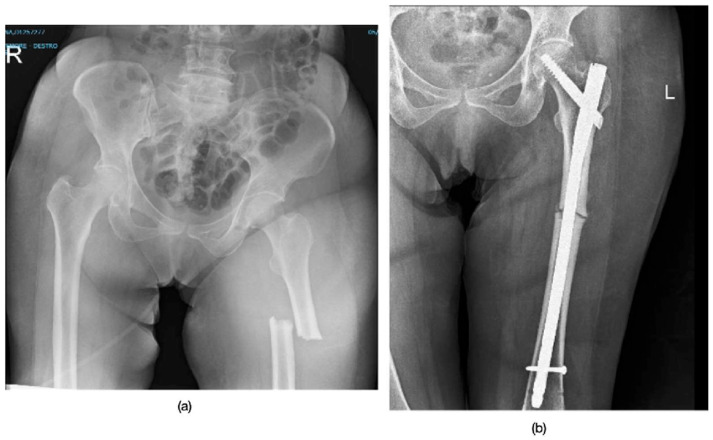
Radiographs of Patient 3, who sustained a subtrochanteric atypical femoral fracture. (a) The pre-operative X-ray explicitly shows the key diagnostic features of an AFF: the fracture originates from the lateral cortex, has a transverse configuration with minimal comminution, and displays localized periosteal thickening of the lateral cortex (beaking). (b) The post-operative image shows fracture stabilization with a long intramedullary nail.
